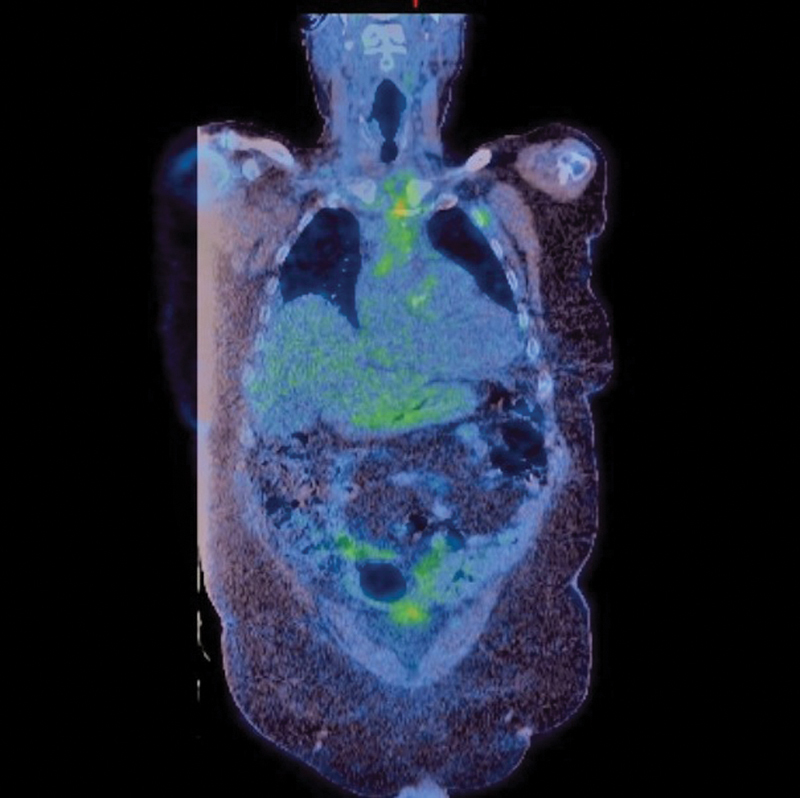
A postoperative half body fluorodeoxyglucose (FDG)-positron emission tomography scan demonstrates aortic valve and ascending aorta replacement with expected postsurgical change, with FDG avid mediastinal fat stranding and no convincing elevated FDG uptake in the wall of the native thoracic or abdominal aorta.
